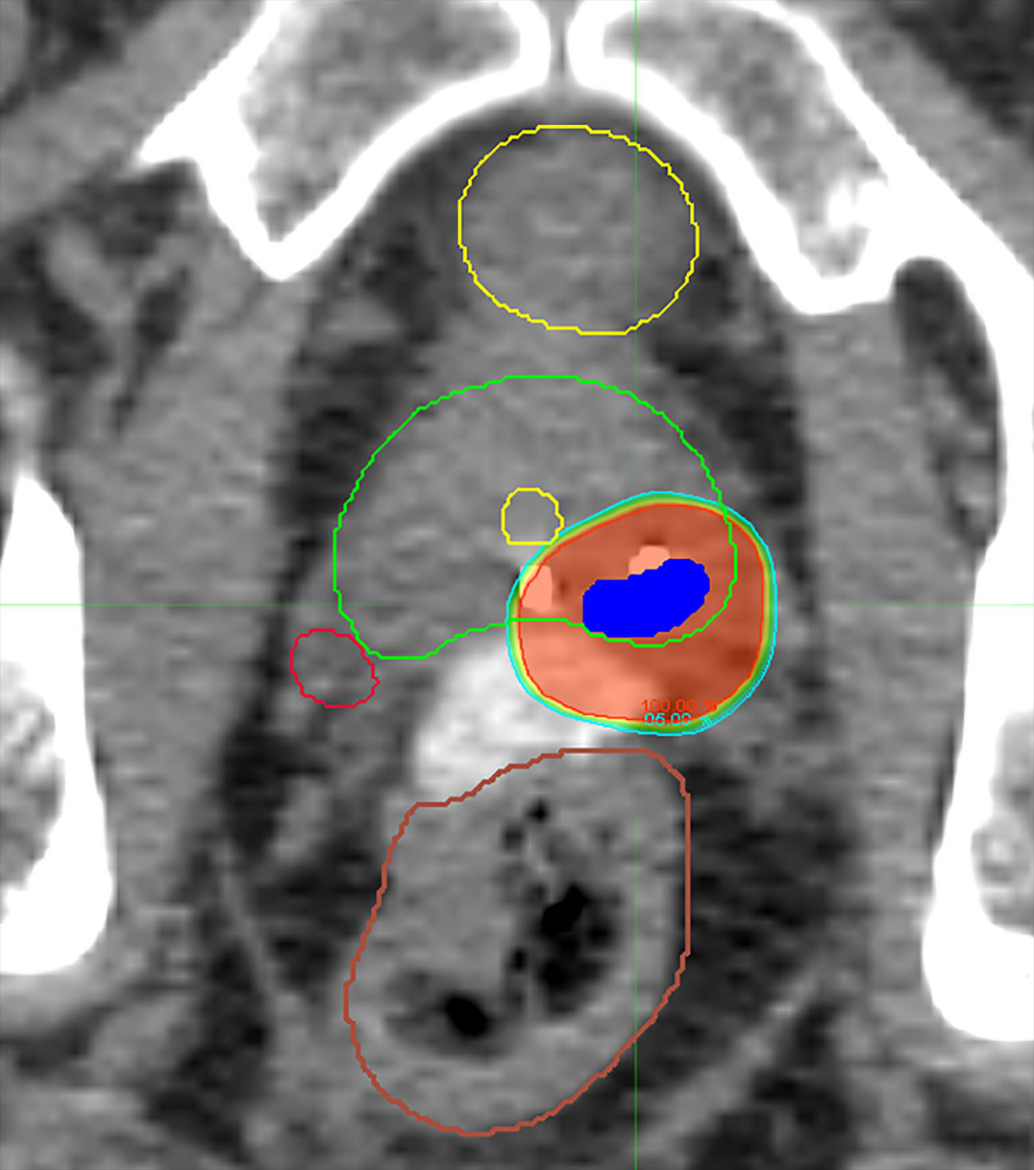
Representative axial CT slice with isodose lines from a delivered f-SBRT plan demonstrating sparing of the uninvolved prostate gland, bladder, rectum, urethra, and contralateral neurovascular bundle.
